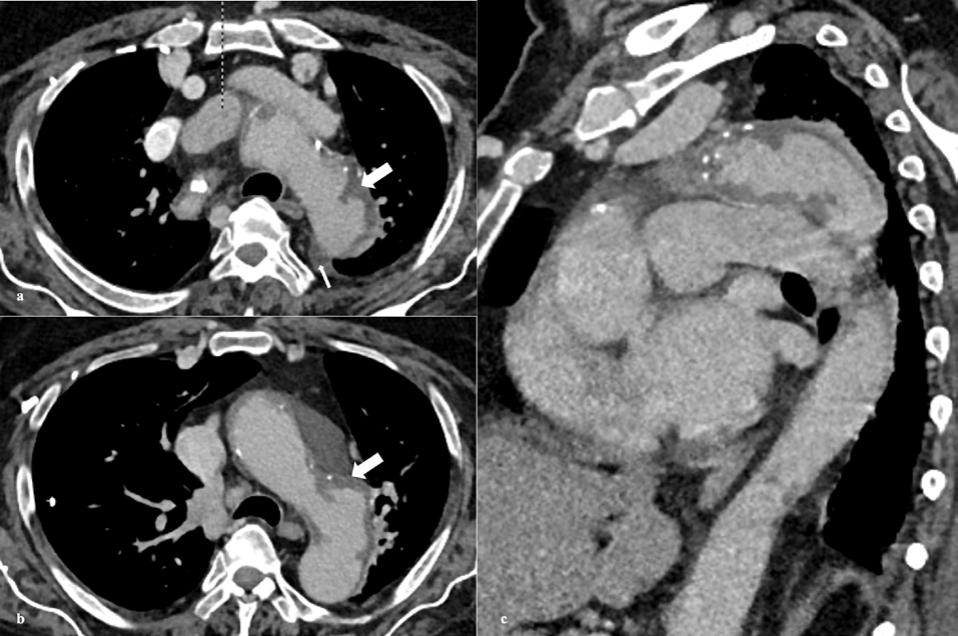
The conventional angiographic and CTA appearance of PAU is similar to a gastric or duodenal ulcer seen in barium studies.^6,13,17^ PAU usually does not extend beyond the aortic contour, latter being suspicious for rupture, associated hematoma or Subintimal pseudoaneurysm formation.^16^ Magnetic resonance imaging (MRI) appearance is akin to the area of flow void showing flow-related enhancement on time-of-flight sequence. Increasing the TE would further enhance the detection of sluggish flow in ulcer crater.^12^ Nonfat sequences were technically less demanding and more accurate than fat saturation sequences and even CTA.
